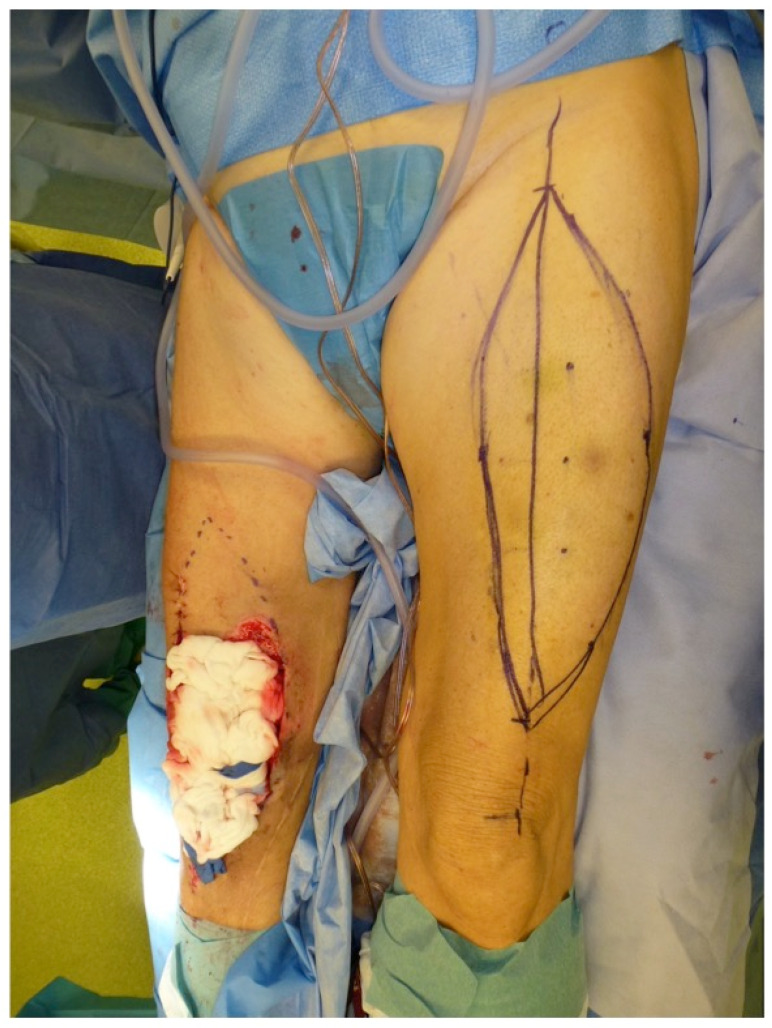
Free ALT with FL: Intraoperative markings to raise the flap to cover the defect around the right knee. The marked dots indicate the skin perforators (identified with a Doppler) off the descending branch of the lateral femoral circumflex artery. Note the planned resection of intact skin proximal to the defect of the right knee to allow flap inset (Table 1: Patient No. 1; additional Figures 3, 10).
